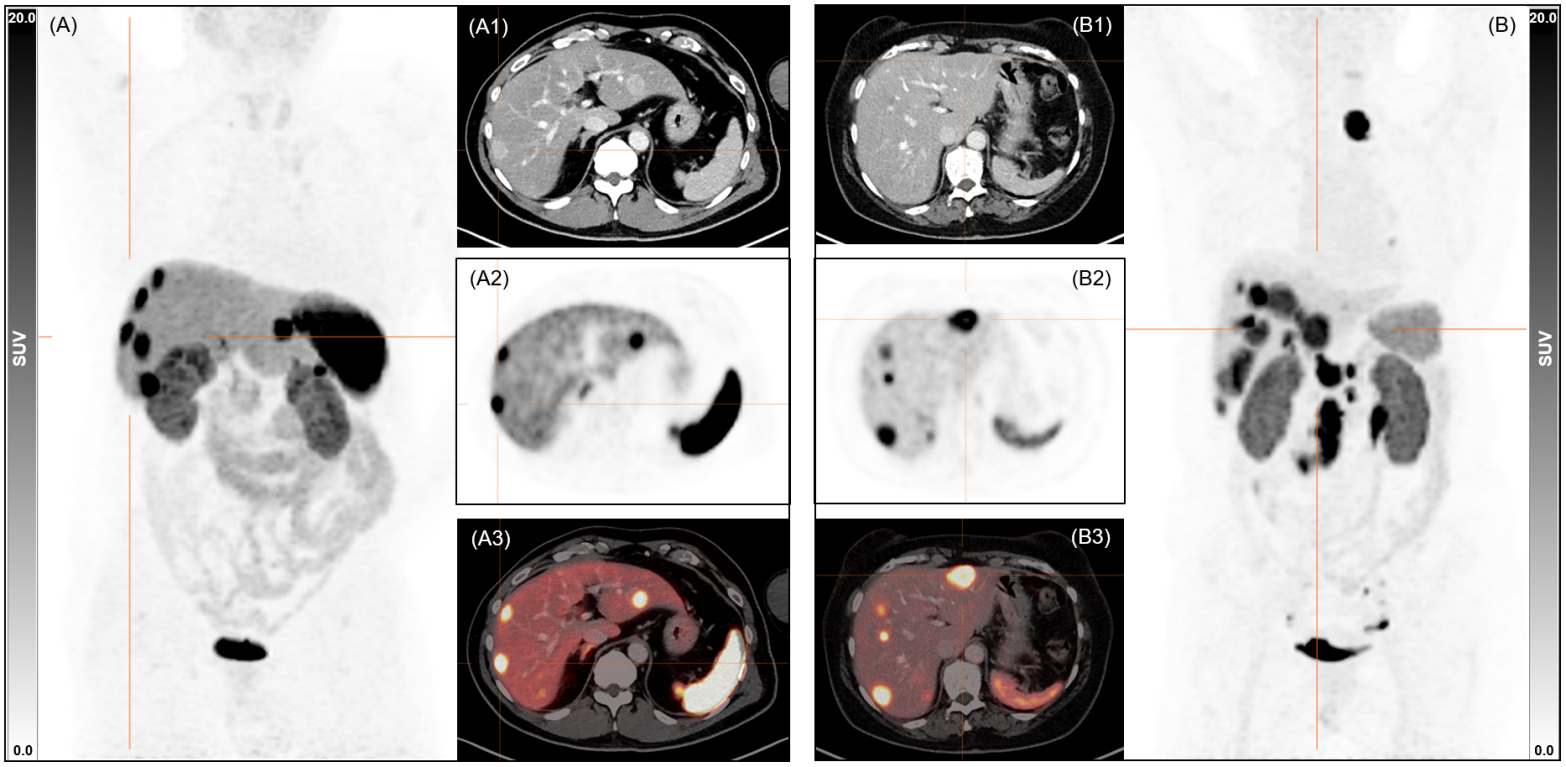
Exemplary maximum intensity projections images and axial sections (1 CT, 2 PET, 3 fused PET/CT) from patients (A) without SSA treatment (male, 59 y, 81 kg, 245 MBq, 89 min p.i., creatinine 1.0 mg/dl, liver SUV_mean_ 8.3, spleen SUV_mean_ 28.0, blood pool SUV_mean_ 1.3) and (B) undergoing SSA treatment (female, 71 y, 64 kg, 281 MBq, 93 min p.i., creatinine 0.7 mg/dl, liver SUV_mean_ 4.1, spleen SUV_mean_ 10.9, blood pool SUV_mean_ 1.6). SUV, standardized uptake value; SSA, somatostatin analogue; MBq, Megabecquerel.
